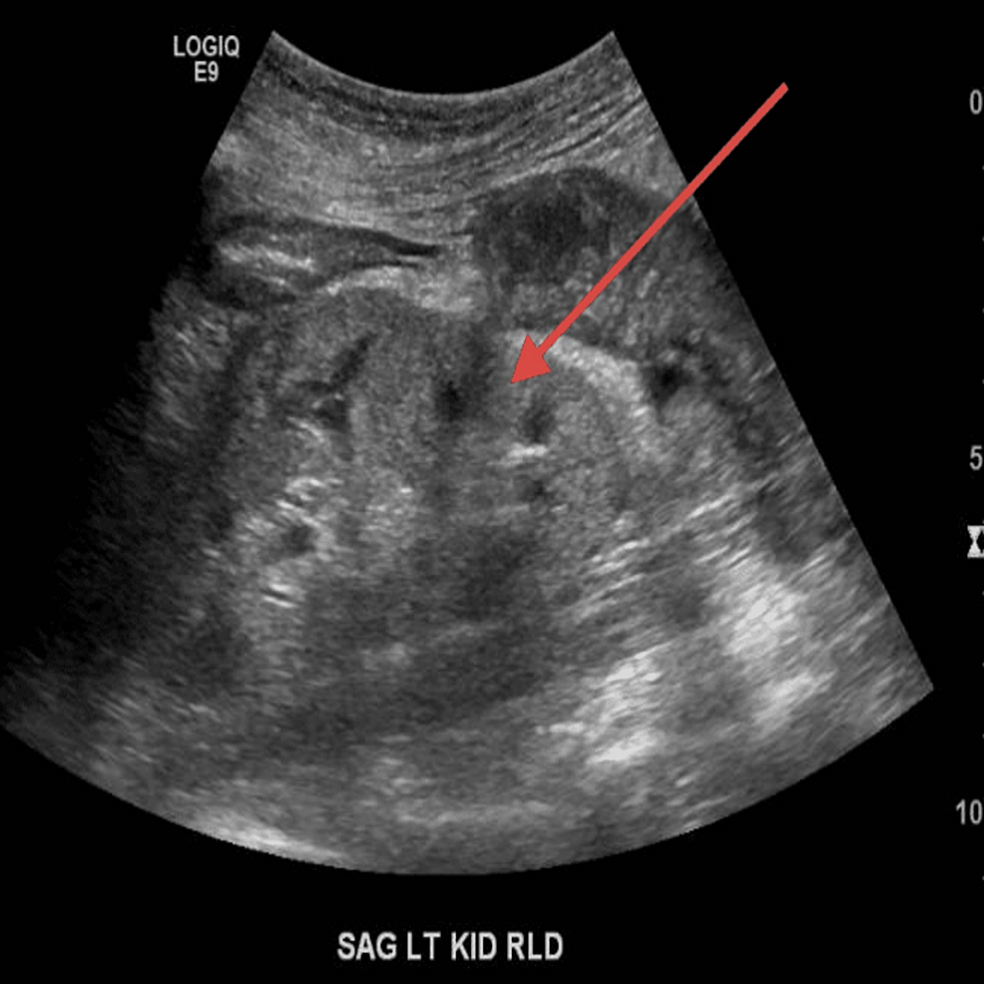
Ultrasound image of the left kidney Ultrasound of the left kidney showing echogenic parenchyma (red arrow).
